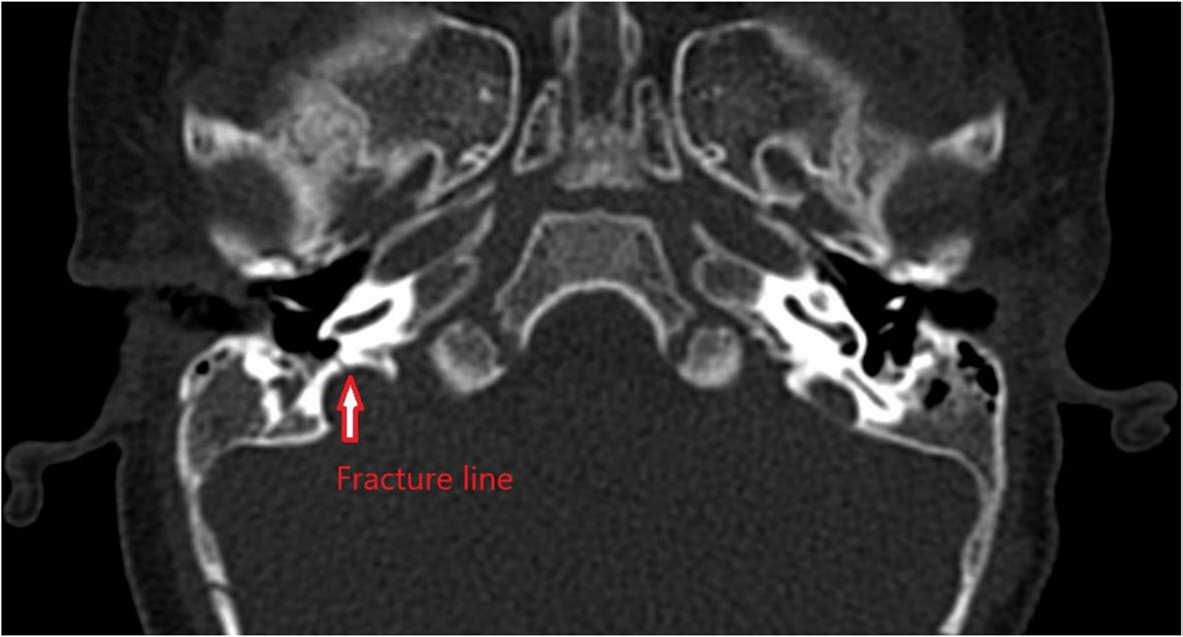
Axial slide of a Computed Tomography scan of the temporal bone without contrast. Axial slide of a Computed Tomography scan of the temporal bone without contrast demonstrating a right transverse temporal bone fracture (red arrow)
